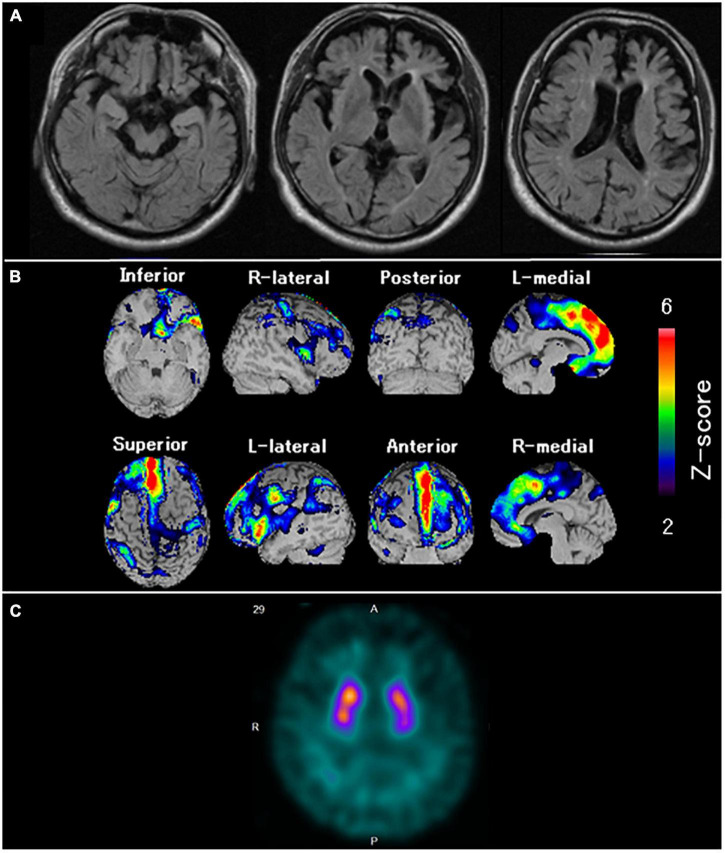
Neuroradiological findings in Case 4. (A) Magnetic resonance imaging shows marked bilateral frontal lobe atrophy, predominant on the left side. (B) Brain perfusion single photon emission computed tomography (SPECT) easy Z-score Imaging System analysis (FUJIFILM Toyama Chemical Co., Ltd., Tokyo, Japan) shows significant bilateral medial frontal lobe hypoperfusion. The color scale for the Z-score is shown on the right side of the figure. Presented in color if the Z-score was > 2. (C) Dopamine transporter (DAT) SPECT shows reduced DAT availability in the bilateral striatum, predominant on the left side.
